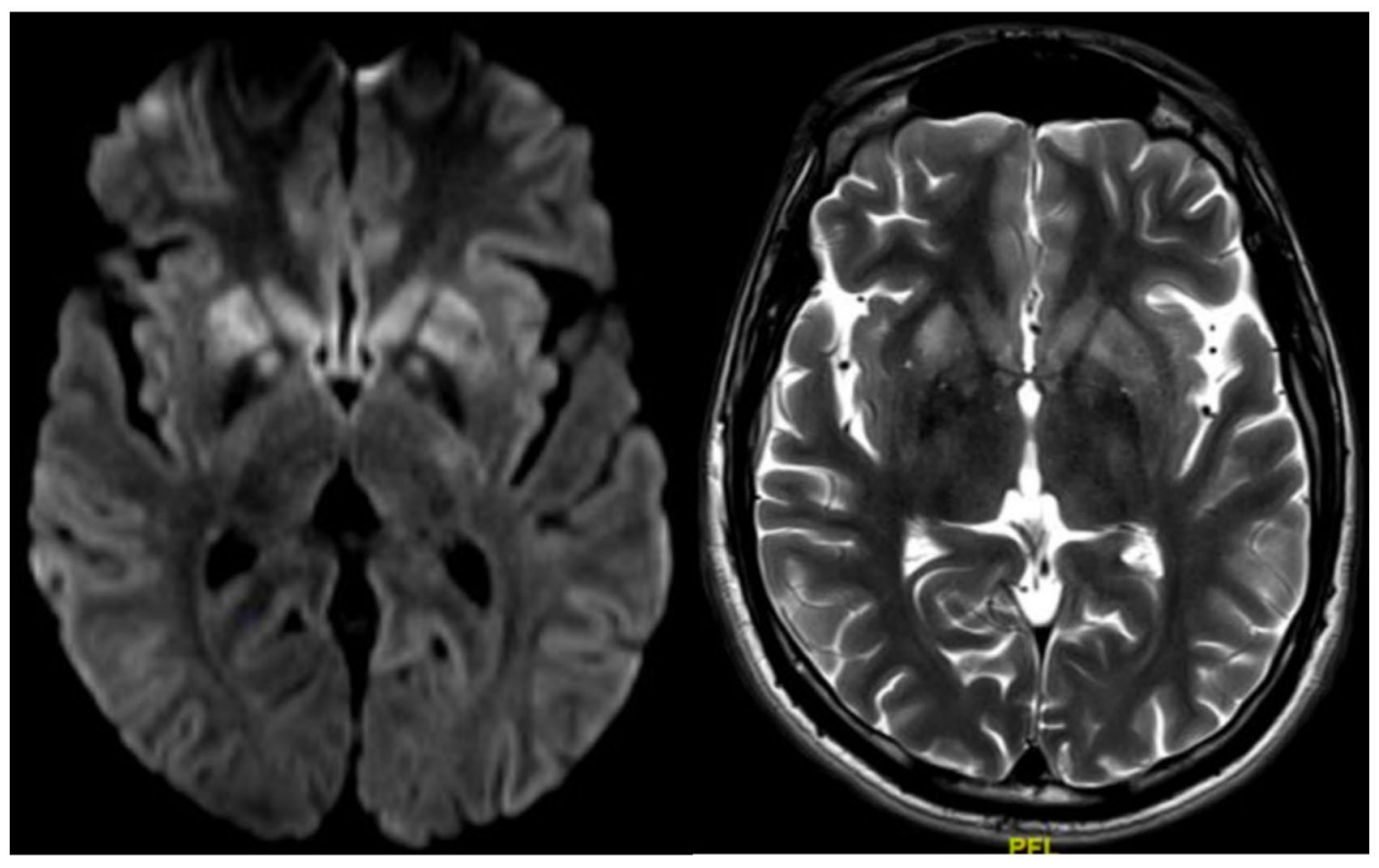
Case 2 MRI: bilateral basal ganglia and substantia nigra hyperintensities (T2, Right; DWI, Left).
